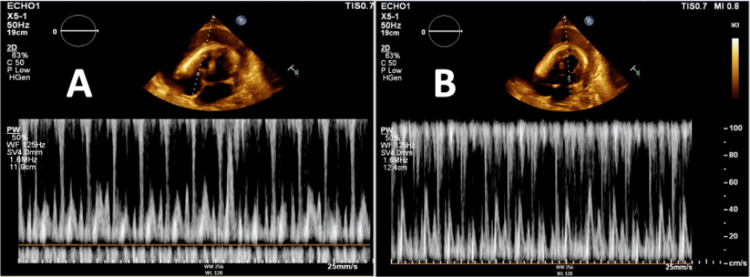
Transthoracic echocardiogram showing tricuspid and mitral valve inflow patterns (A) Respiratory variability in tricuspid inflow velocity less than 40%. (B) Respiratory variability in mitral valve inflow velocity greater than 25% consistent with impending tamponade.
